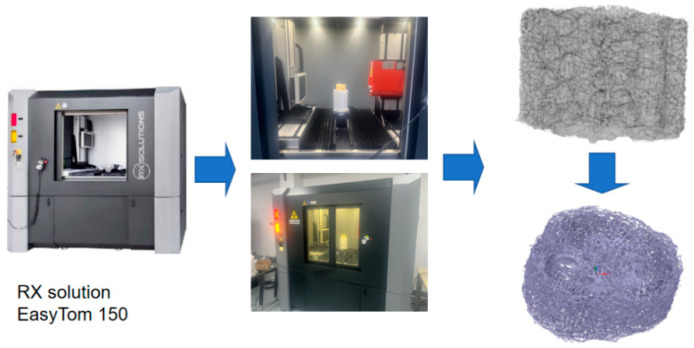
Industrial CT scan modelling.
